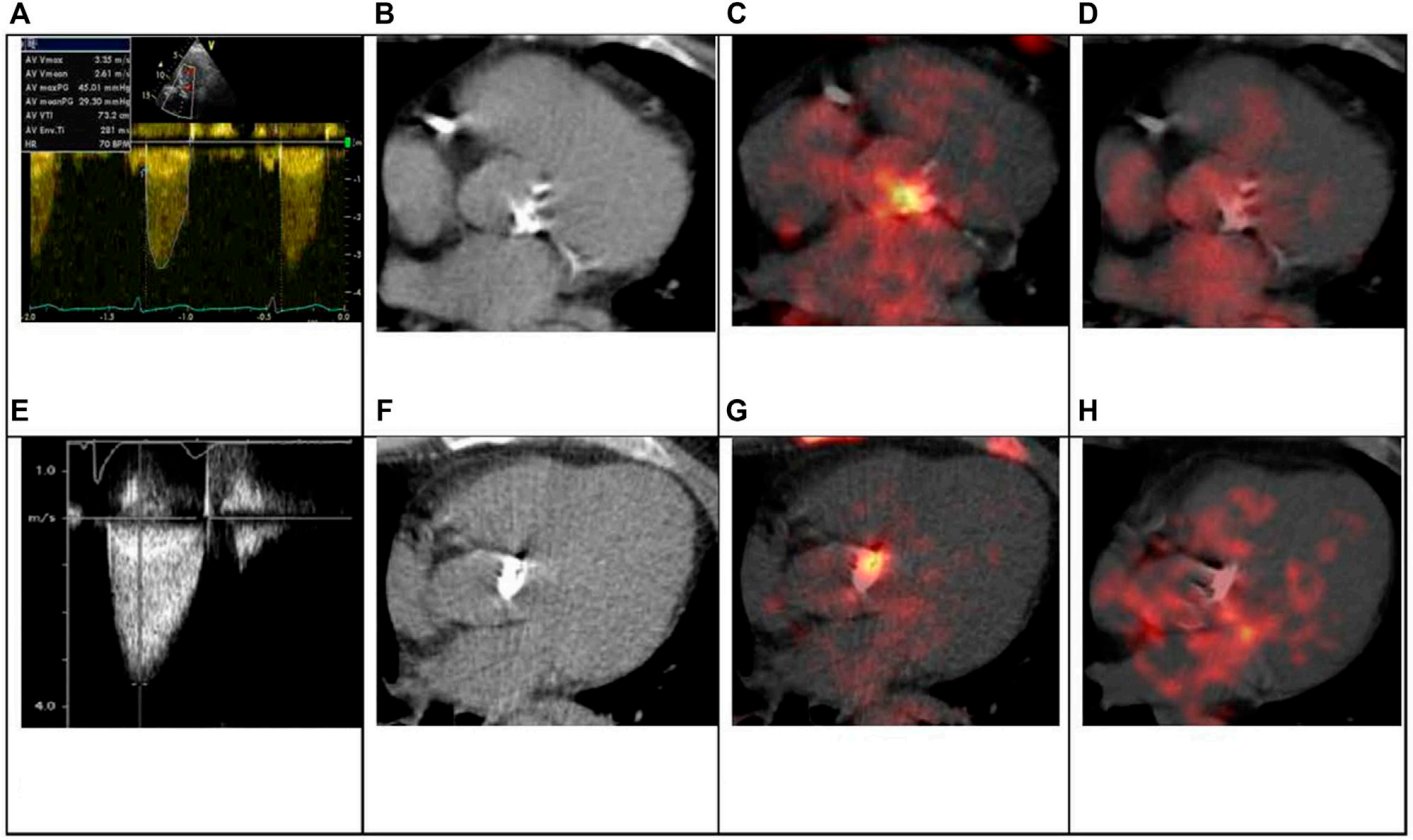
TAV and BAV patient with moderate AS. 64-years old asymptomatic patient with TAV. Echo parameters: Vmax 3.35 m/s, mean gradient 29 mmHg (A). CT revealed and severe aortic valve calcification: Agatston Score 3139 AU (B). ^18^F-NaF PET/CT demonstrated marked accumulation of the radiotracer: ^18^F-NaF TBR max 1.91 (C). ^18^F-FDG valve accumulation was not significant, ^18^F-FDG TBR max 1.05 (D). 2 years hence symptoms manifested, Vmax increased to 4.2 m/s and aortic valve replacement was performed. 51-years old patient with BAV. Echo parameters: Vmax 3.4 m/s, mean gradient 30 mmHg (E). CT revealed and severe aortic valve calcification: Agatston Score 4769 AU (F). ^18^F-NaF PET/CT demonstrated significant accumulation of the radiotracer: ^18^F-NaF TBR max 2.58 (G). ^18^F-FDG valve uptake was not increased, ^18^F-FDG TBR max 1.17 (H). There was no clinical or hemodynamic progression registered during 4-years follow up.
